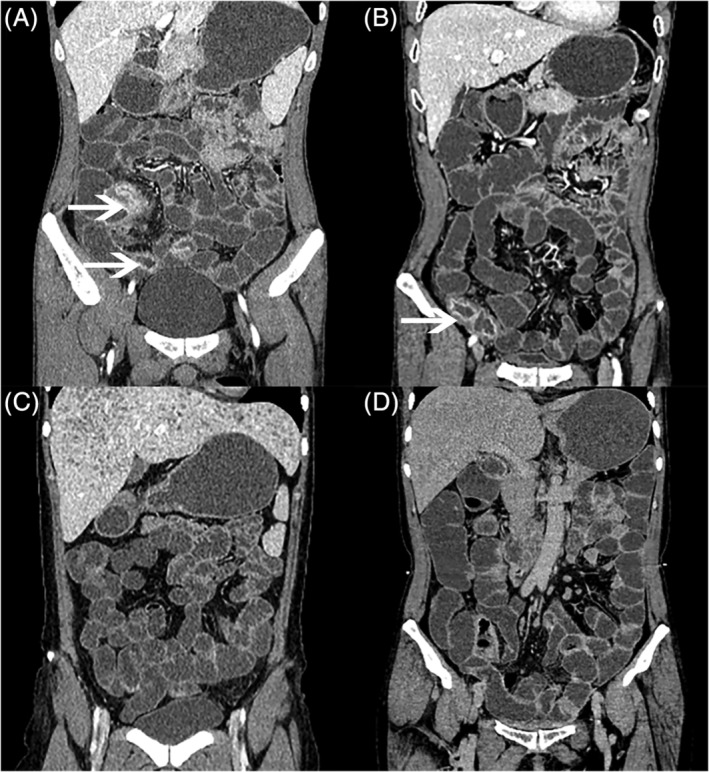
Coronal computed tomography enterography images. A and B, two patients with small bowel diseases; C and D, two patients without intestinal abnormalities. A and C were prepared with mannitol, and B and D were prepared with polyethylene glycol (PEG), respectively. A, B, Both contrast agents clearly depicted mural hyperenhancement, thickening and perienteric inflammatory changes (arrows)
